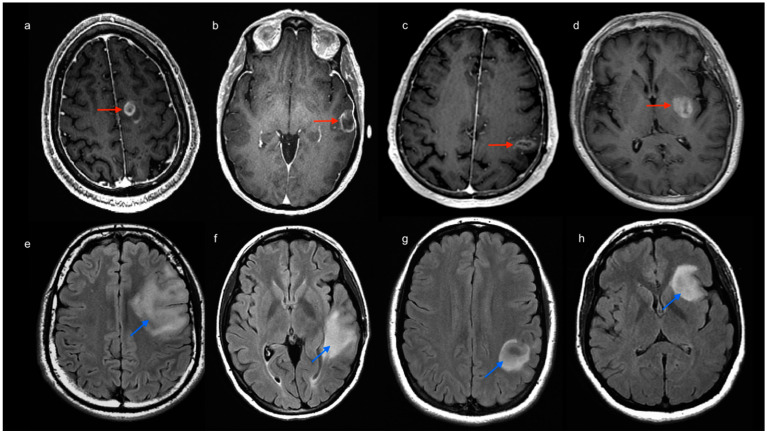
Images above: post-contrast 3D T1-weighted MR scans of four patients with high-grade glioma (image a–d, red arrows). Tumor location was labeled to account for involved lobes as follows: frontal (a), temporal (b), parietal (c) and insular involvement (d). Images below: FLAIR-weighted MR scans of four patients with low-grade glioma (image e–h, light blue arrows). Tumor location was labeled to account for involved lobes as follows: frontal (e), temporal (f), parietal (g) and insular involvement (h).
